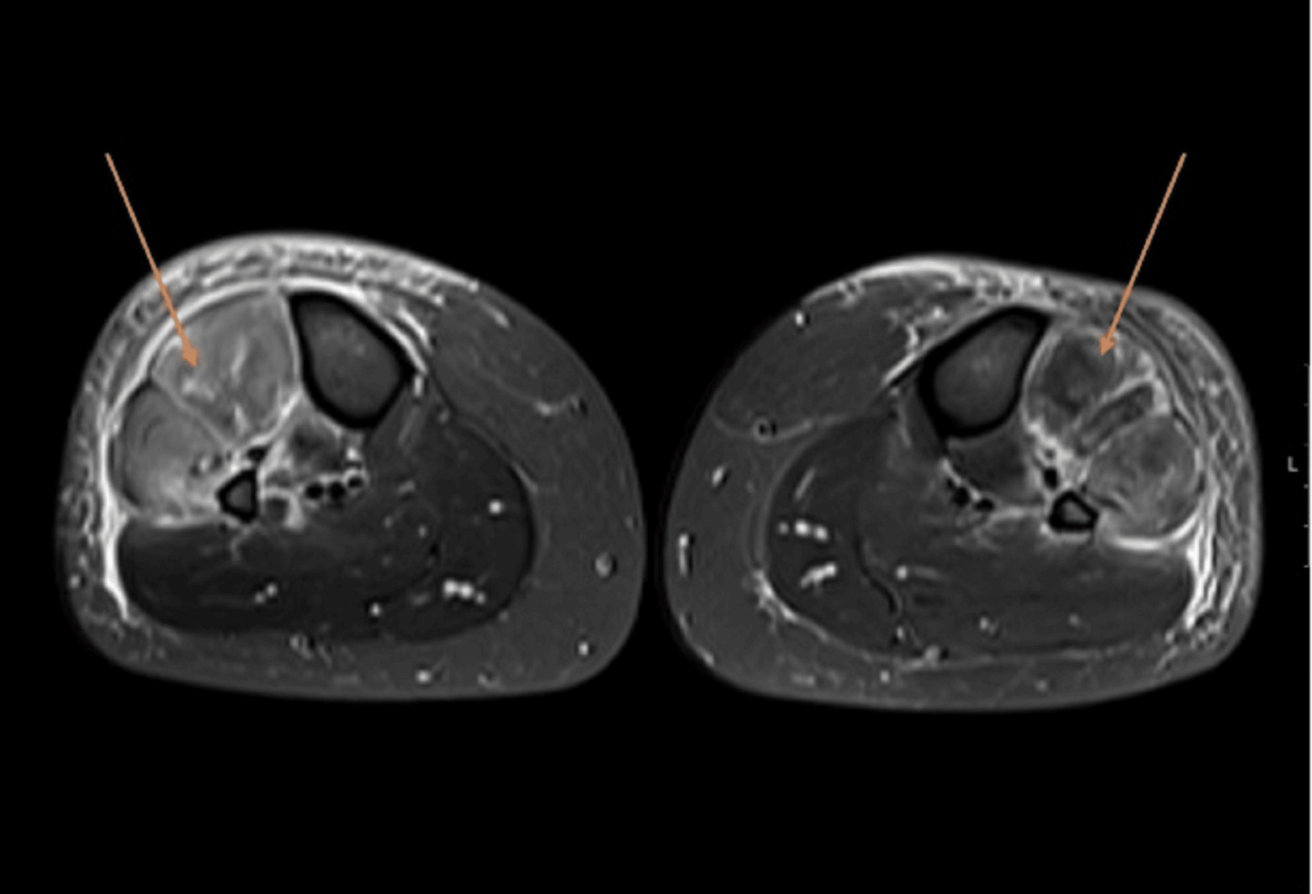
MRI calves axial view. T2 hyperintensity consistent with muscle edema (arrows). MRI, magnetic resonance imaging
